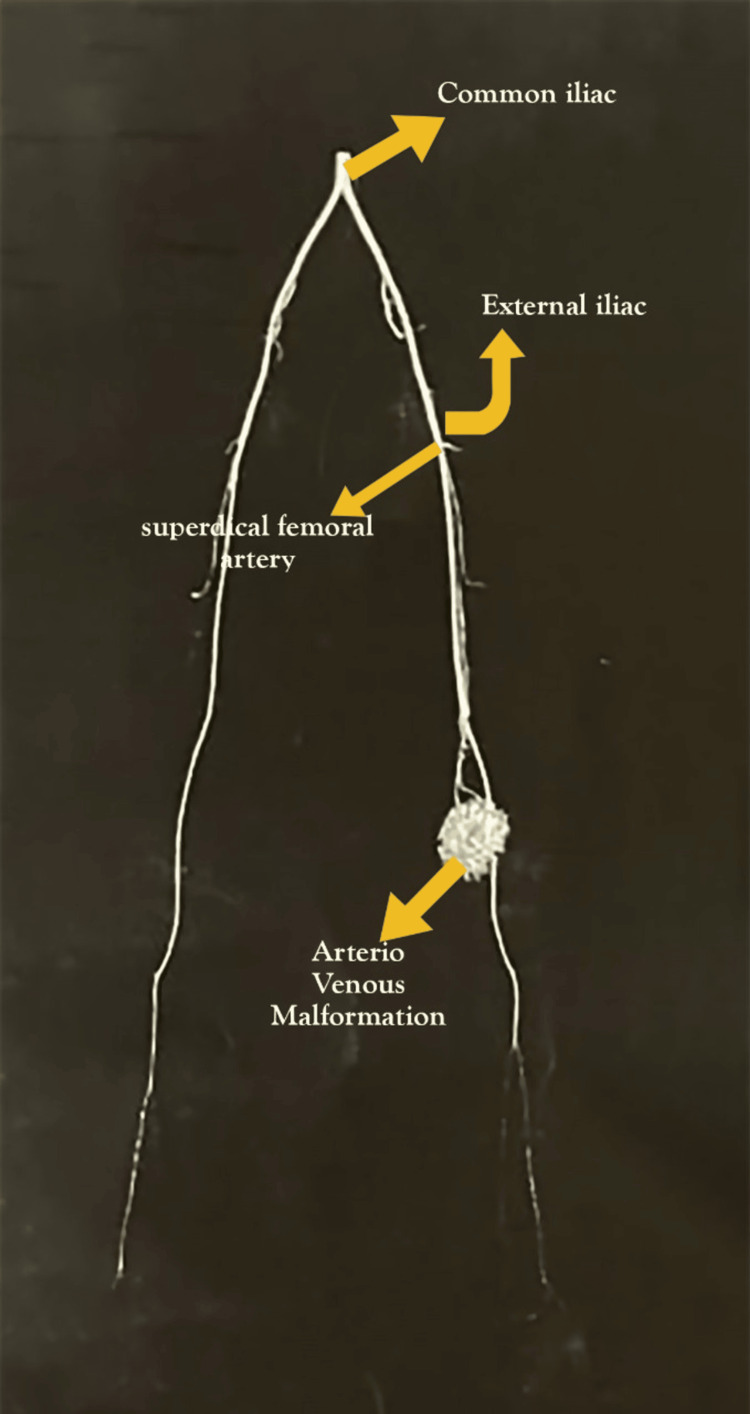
CT lower limb angiography CT bilateral lower limb angiography revealed a well-defined, avidly enhancing subcutaneous lesion measuring 4.5×1.3×3.9 cm on the medial aspect of the left knee. Enlarged muscular branches of the superficial femoral artery were supplying the lesion, with the largest branch arising approximately 7.7 cm proximal to the knee. Multiple venous channels were observed draining into the superficial femoral vein above the knee, with early enhancement noted in the arterial phase. CT: computed tomography
